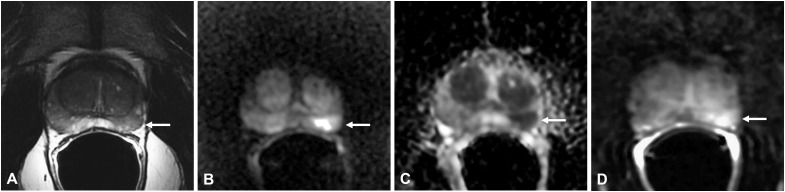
Non-specific granulomatous prostatitis in a 56-year-old patient with a PSA value of 6 ng/ml. The axial T2-weighted sequence shows a hypointense nodule in the left mid-peripheral zone (PZ) (arrow in A) with high hyperintensity on the DWI image (arrow in B), marked hypointensity on the ADC map (arrow in C), and high contrast enhancement on the DCE image (arrow in D).
